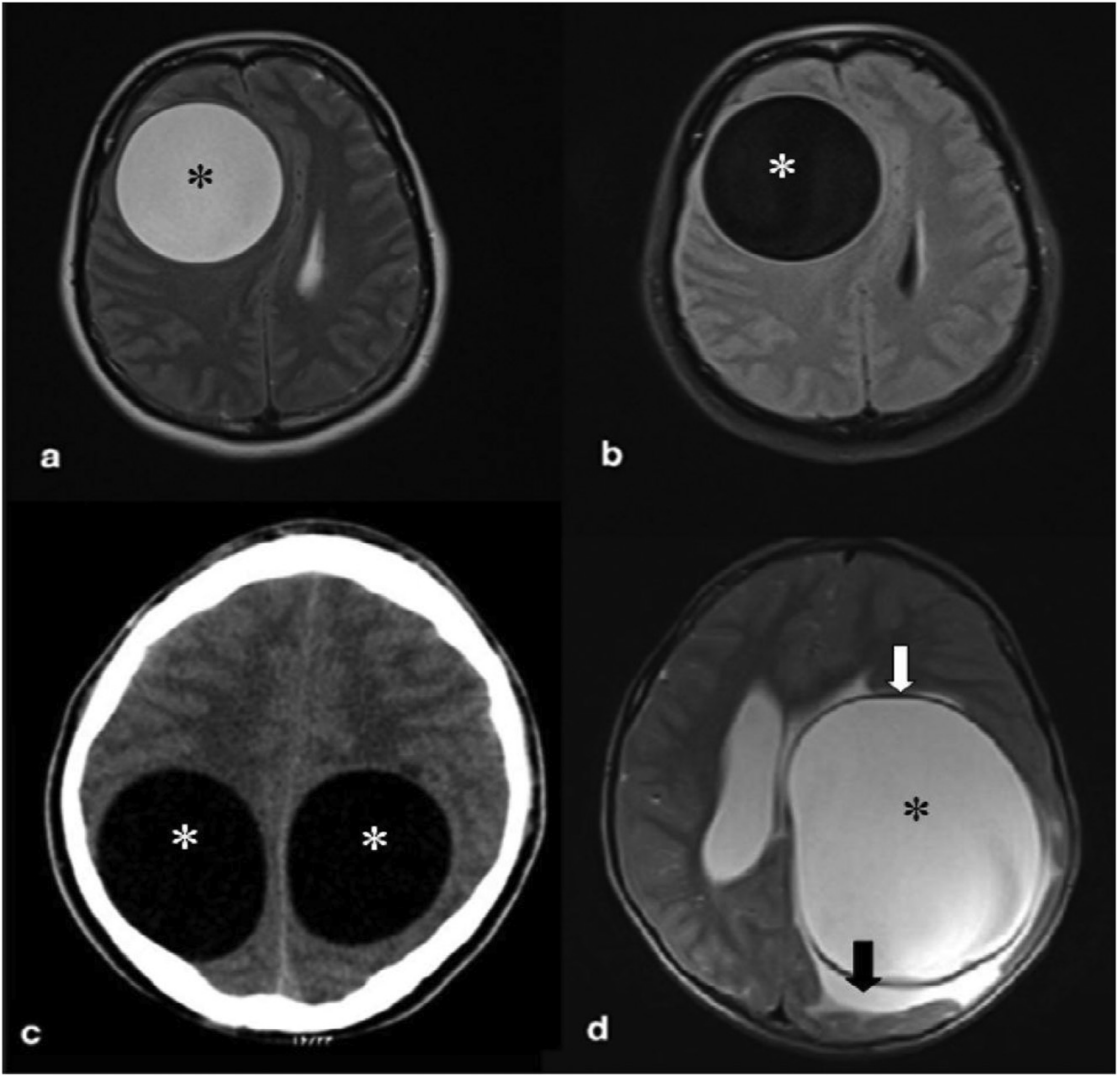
Axial T2-weighted (a) and FLAIR (b) MRI in a young man show a well-defined, round cystic lesion (Asterisk) in the right hemisphere. Axial brain CT scan in a 12-year-old child (c) shows two well-defined cystic lesions (asterisks) in the parietal lobes that were proved to be hydatid cysts after surgical removal. Axial T2-weighted brain MRI (d) in a different patient shows a large intracranial cyst (Asterisk) with low-signal wall (white arrow) and minimal peripheral edema (black arrow). (Photos are from our own patient(s) and they had not been published previously elsewhere.).
